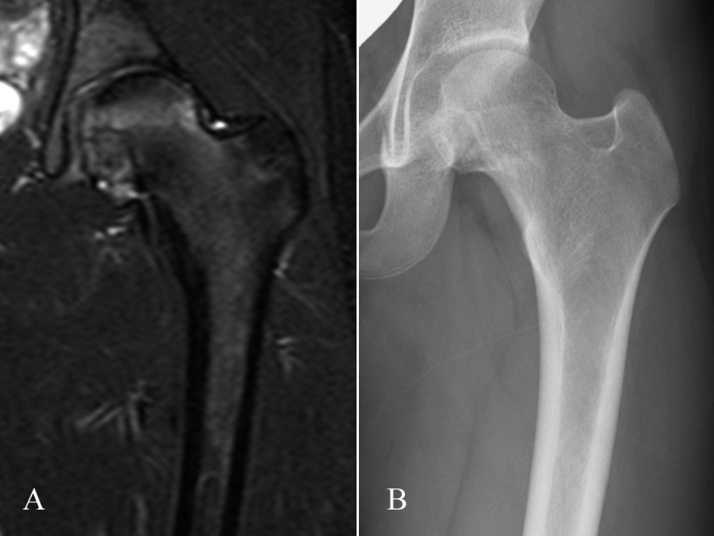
A STIR MR image at 18 months after surgery (A) and a radiograph at 31 months after surgery (B).
